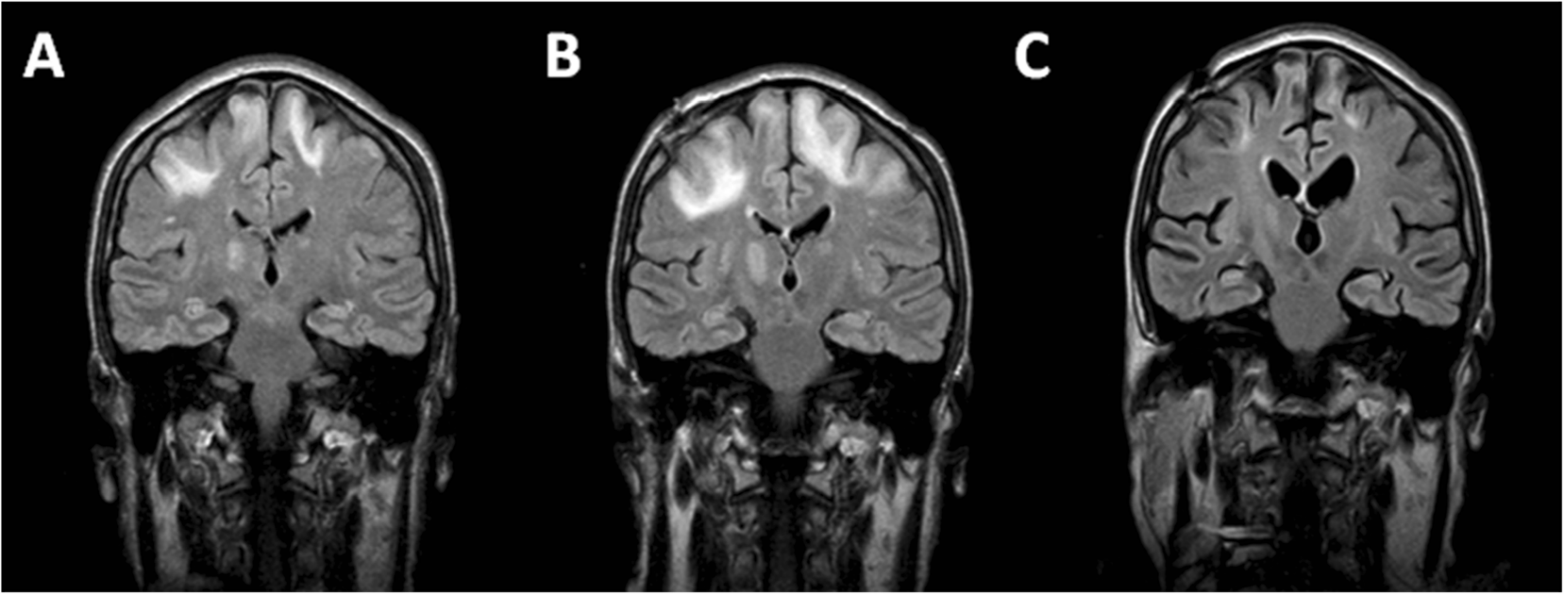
Fluid-attenuated inversion recovery (FLAIR) images in the affected regions. A: bilateral hyperintensity in the white matter involving the parietal and occipital lobules and the internal capsule. B: Increment in the extension and intensity of the parietal lesion, one month after diagnosis. C: Complete resolution of the lesion, one year after diagnosis.
